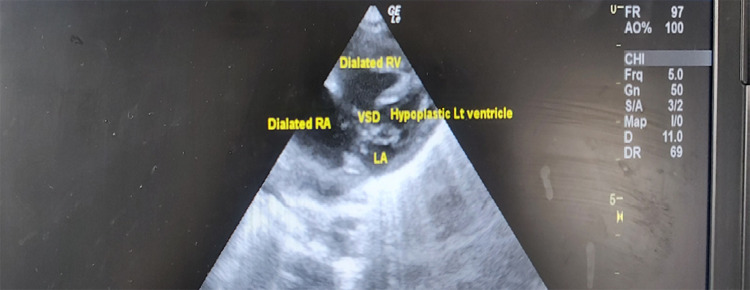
2D echocardiography subcostal view showing dilated RV and RA, large VSD with hypoplastic LV at 23 hours of life.
